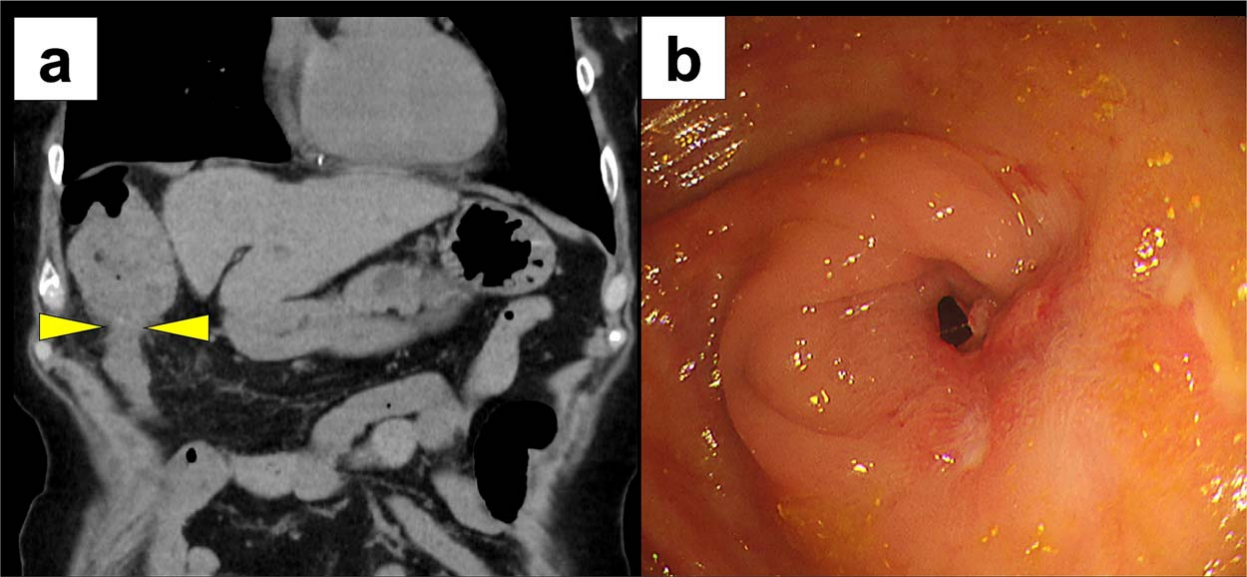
Postoperative imaging studies. (a) CT scan showing stenosis of the transverse colon with massive fecal impaction in the ascending colon (arrow) and massive fecal impaction in the ascending colon. (b) Lower gastrointestinal endoscopy revealing severe stenosis at the hepatic flexure of the transverse colon.
